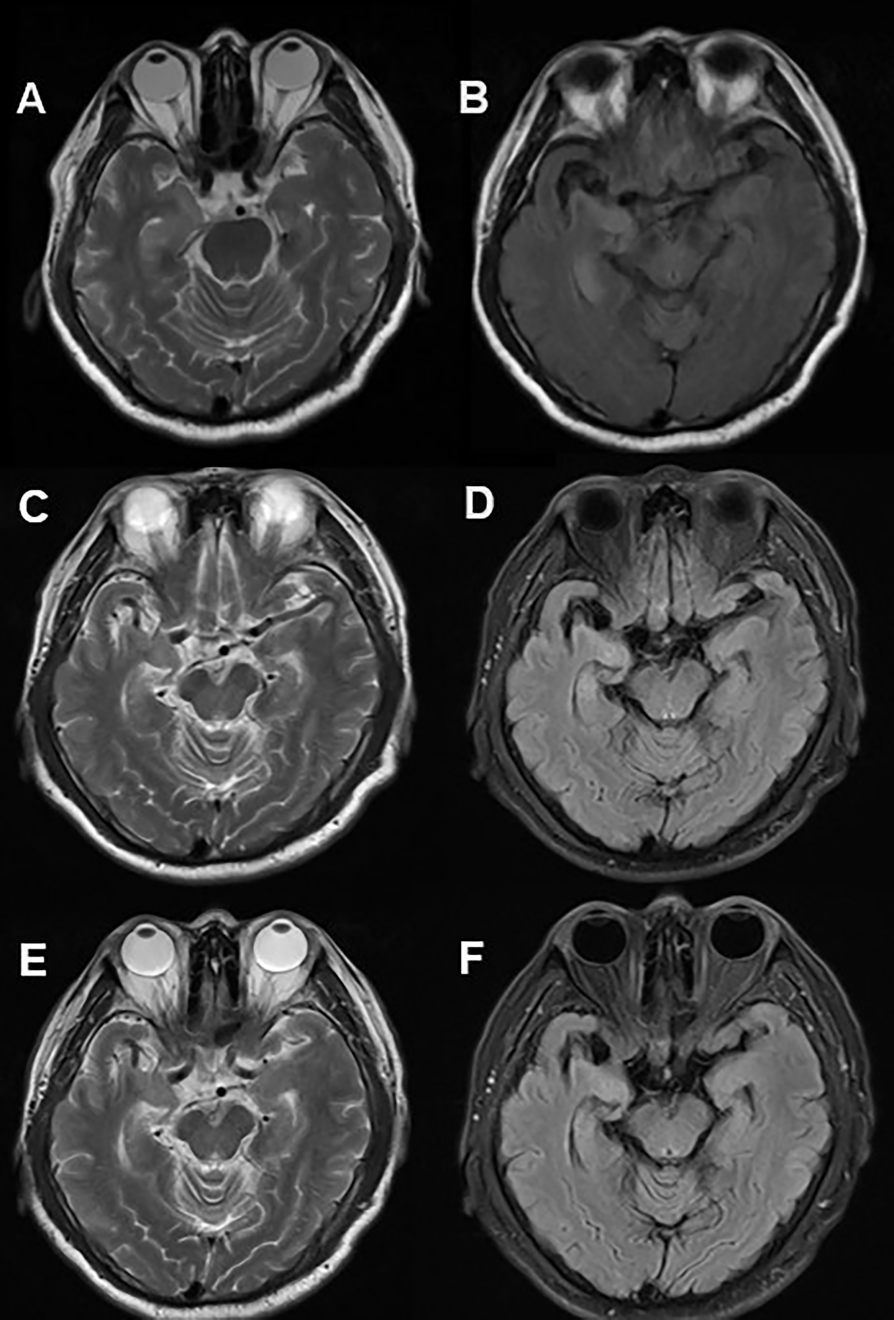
Cranial MRI in Case 1. Axial T2 weighted (A) and FLAIR (B) sequences showed hyper-intensity in the right hippocampus. 20 months after immunotherapy, a control cranial MRI showed a significant reduction of right hippocampus hyper-intensity in T2 (C) weighted and FLAIR (D) sequences. 28 months after immunotherapy, no significant changes contrasted (E, F) with (C, D).
